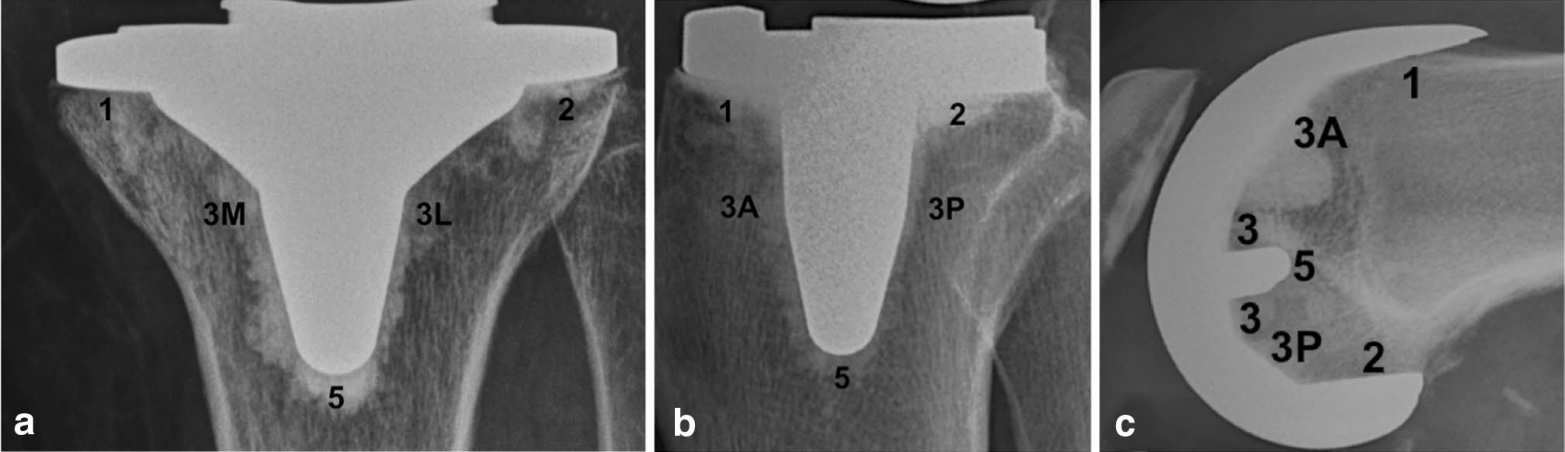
Radiographic evaluation of radiolucent lines according to the “Modern Knee Society Radiographic Evaluation System and Methodology for Total Knee Arthroplasty” [13]: a anterior–posterior radiograph of tibial component (3M: medial aspect of the keel; 3L: lateral aspect of the keel); b lateral radiograph of tibial component (3A: anterior aspect of the keel; 3P: posterior aspect of the keel); c lateral radiograph of femoral component
